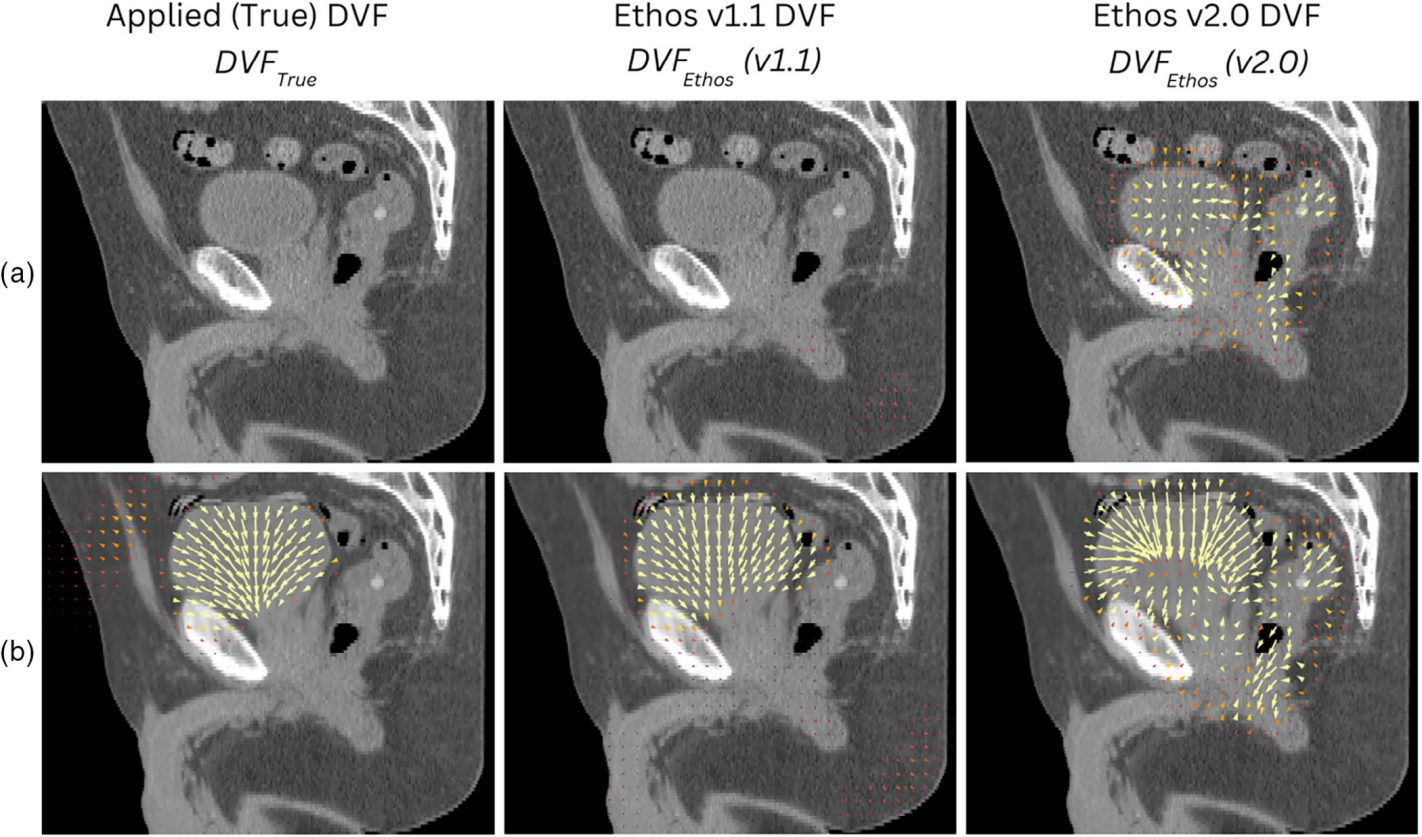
Sagittal visualization of the DVF overlaid on the modified CT. Row A displays DVFs from *Part 2 ‐ Prostate* Fx1, where no deformation was applied to the planning CT. Row B displays DVFs from *Part 2 ‐ Prostate* Fx4, where the bladder was expanded by a large amount. Note, *DVF_True_
